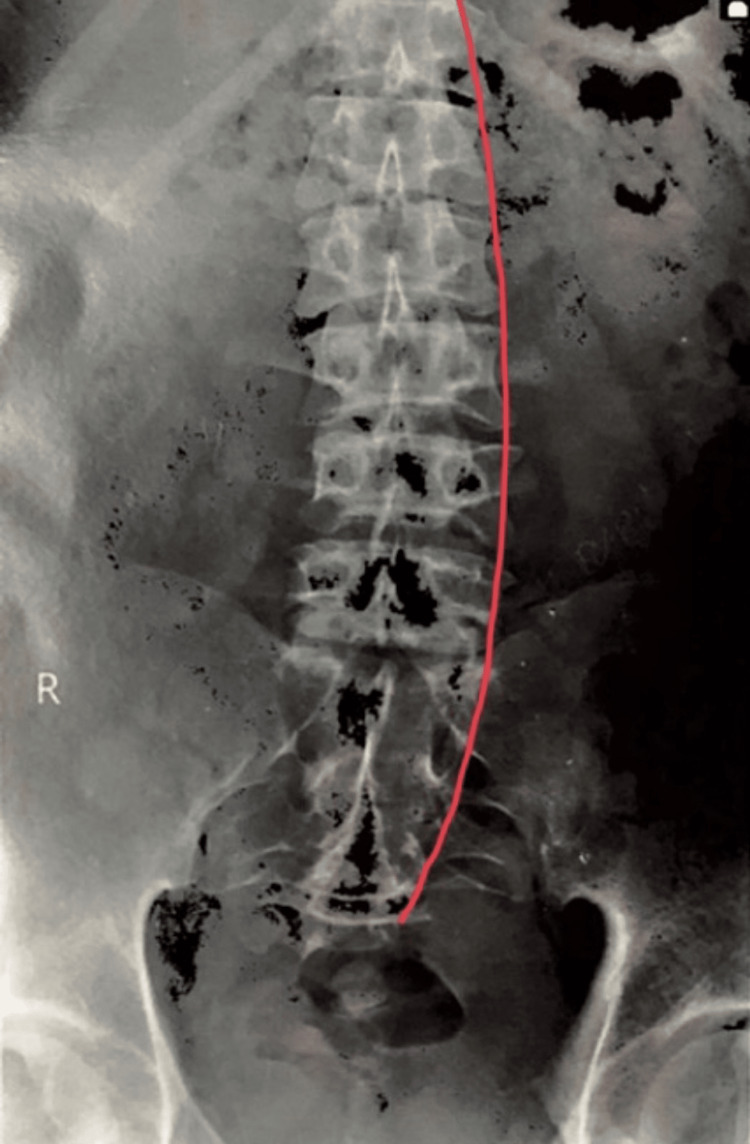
X-Ray shows a slight bent in the vertebra The red line shows a curve in the spine Image Credit: Author: Arpit Jain
